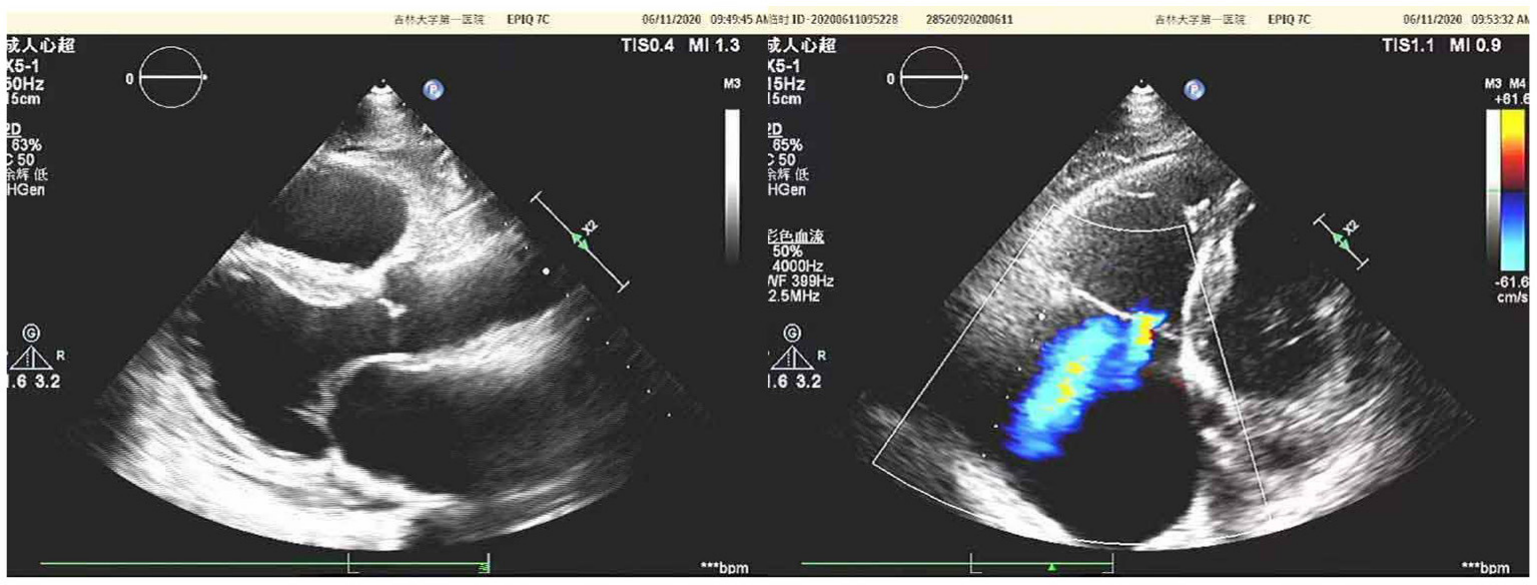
Echocardiographic of the patient's heart [a left ventricular ejection fraction (LVEF) of 46% (M-type Teichholz method), a right ventricular internal diameter of 26 mm, a right atrial diameter of 54 × 64 mm, diffusely attenuated right ventricular wall pulsation, and the tricuspid plane systolic excursion (TAPSE) was 12 mm, and the tricuspid regurgitation was severe].
